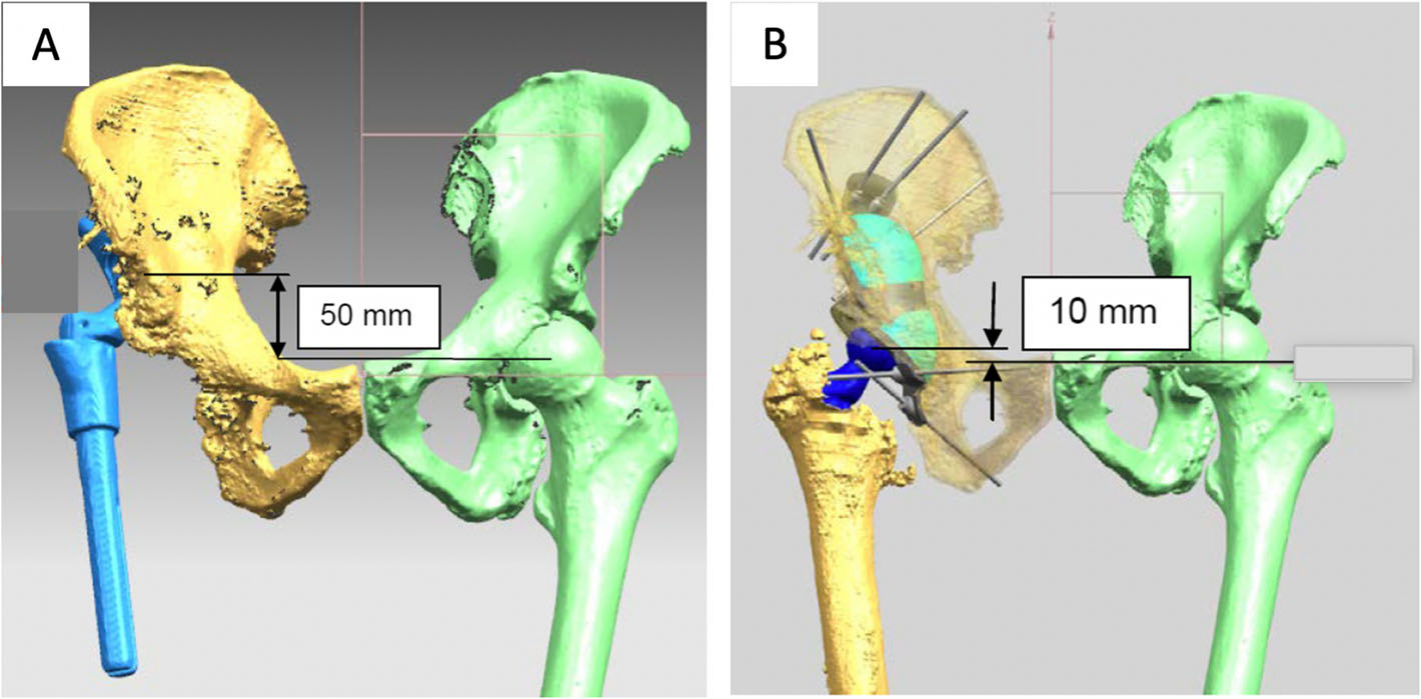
Example of CT surgical plan where complete equalization of left and right centre of rotation was not the goal, a residual 10 mm difference between right and left hip was planned. Decision was made considering the anatomical characteristics of the patients alongside with the design of the bespoke implant to be inserted in the acetabular cavity
